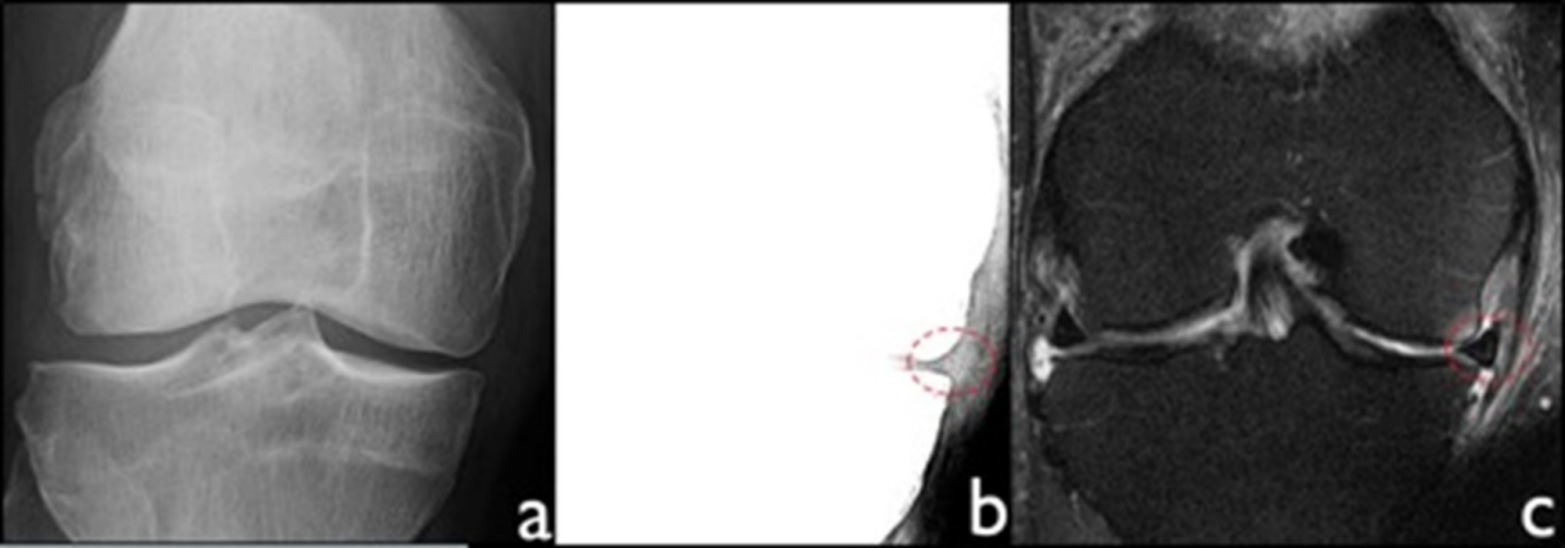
Radiographic appearance of meniscal extrusion. a) AP knee radiograph with standard settings. b) AP knee radiograph with narrow window settings demonstrates medial meniscal extrusion greater than 3 mm as measured by tissue extending beyond the medial tibial plateau, excluding osteophytes. c) Correlative coronal proton density fat saturation MRI confirms presence of extrusion. MRI - magnetic resonance imaging.
